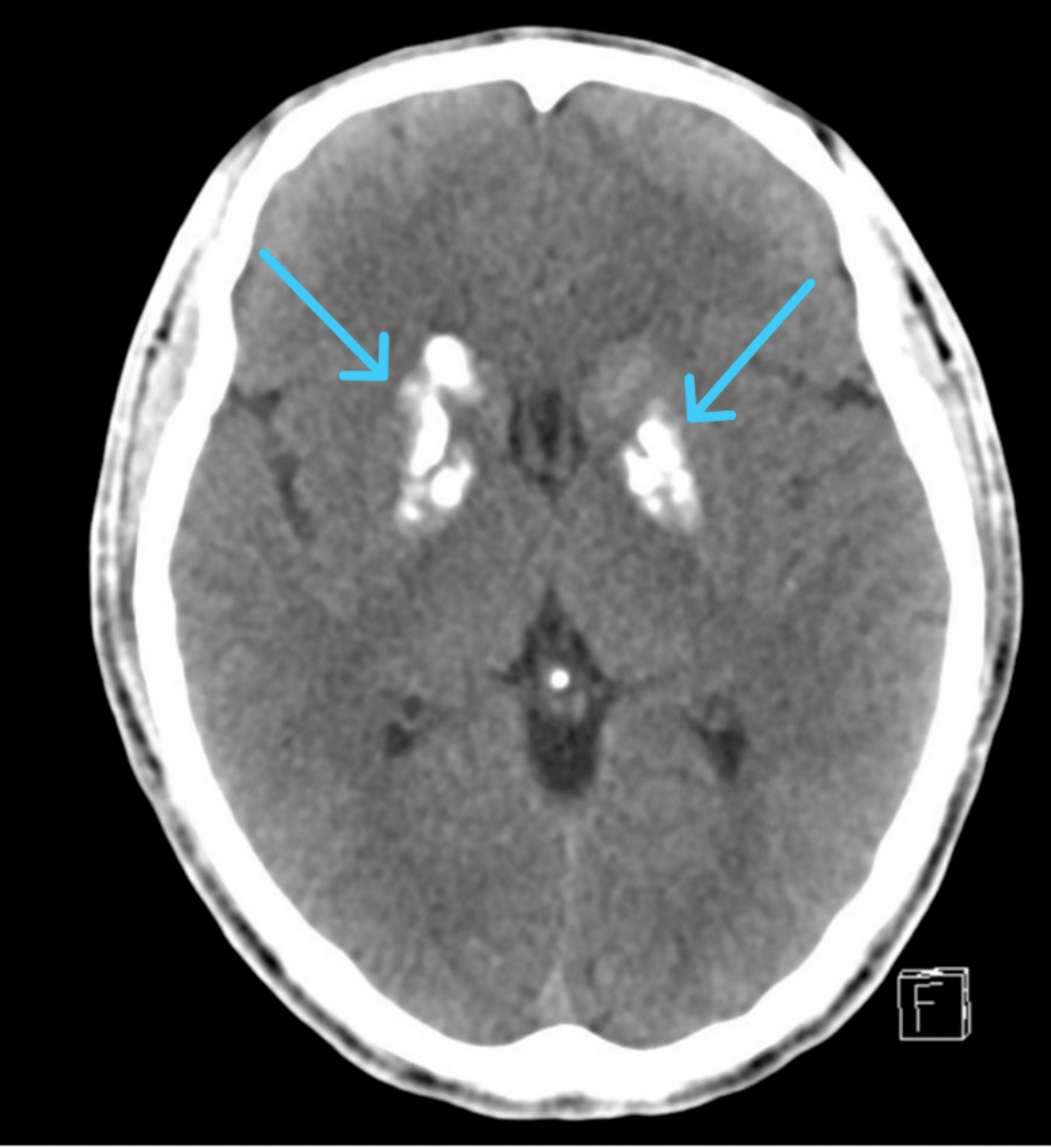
Brain CT scan showing intracranial calcifications in Fahr’s disease/syndrome. Dense bilateral calcifications are seen in the basal ganglia (blue arrows), with additional smaller calcifications in the subcortical frontal white matter, right corona radiata, and right dentate nucleus. These findings are characteristic of Fahr’s disease/syndrome, indicating abnormal calcium deposition across multiple neuroanatomical regions.
